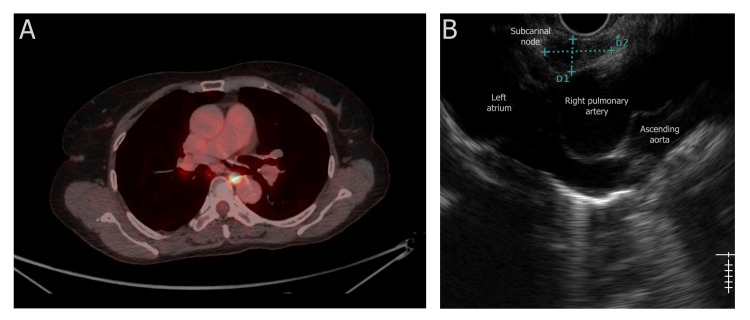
(A) 18F-fluorodeoxyglucose uptake noted in the subcarinal node on PET/CT; (B) endoscopic ultrasound image showing a hypoechoic, well-defined lymph node in station 7
